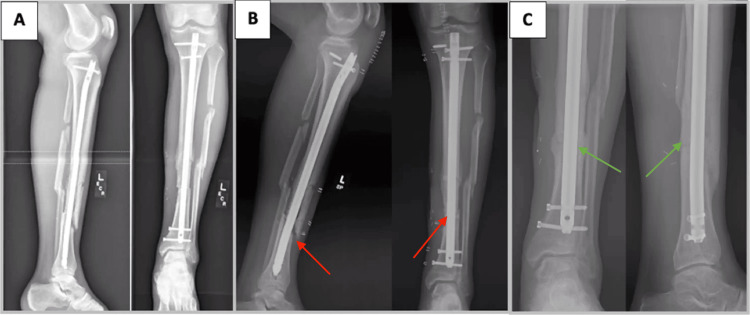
A 56-year-old male smoker with oligotrophic nonunion of a segmental tibial fracture (case 3) A) Radiographs of the patient’s left tibia four months after injury, status post tibia and fibula intramedullary nailing at an outside hospital with soleus flap coverage and skin grafting. B) Radiographs of the left tibia demonstrating persistent nonunion (red arrows) with no callous formation at fracture site six months after index surgery. C) Radiographs demonstrating union (green arrows) approximately one year after exchange nailing performed with adjunctive cortical vent drill tunnels. The patient was pain-free at the former tibial nonunion site.
